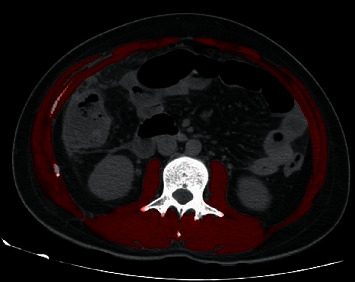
Evaluation of the skeletal muscle mass using a third lumbar computed tomography scan slice. Red: skeletal muscle (including the psoas, paraspinal, and abdominal wall muscles).
